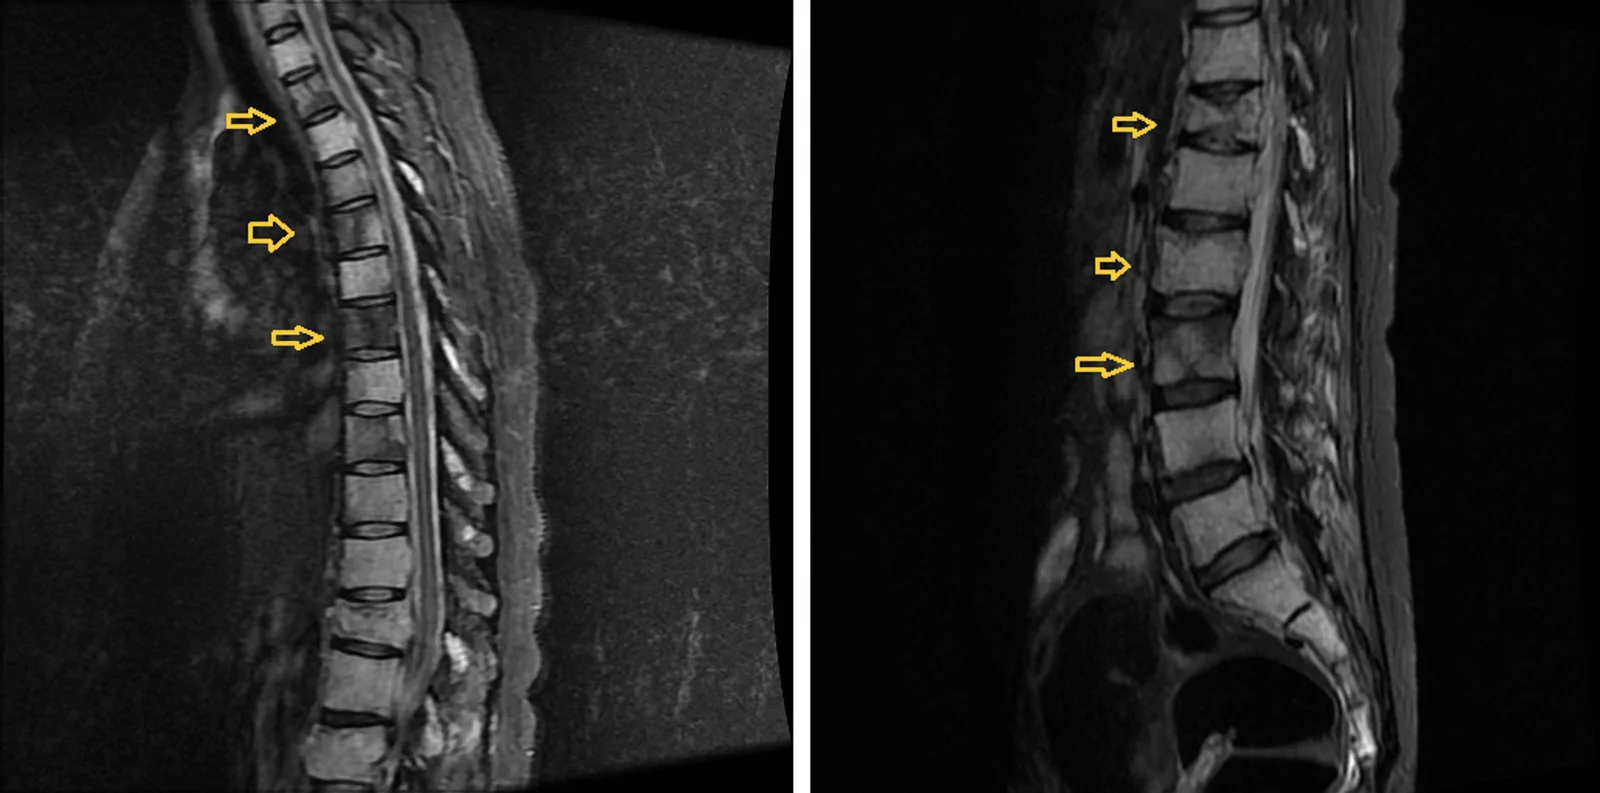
MRI spine suggestive of multiple pathologic compression fractures across the vertebral column due to metastatic involvement (arrows).
